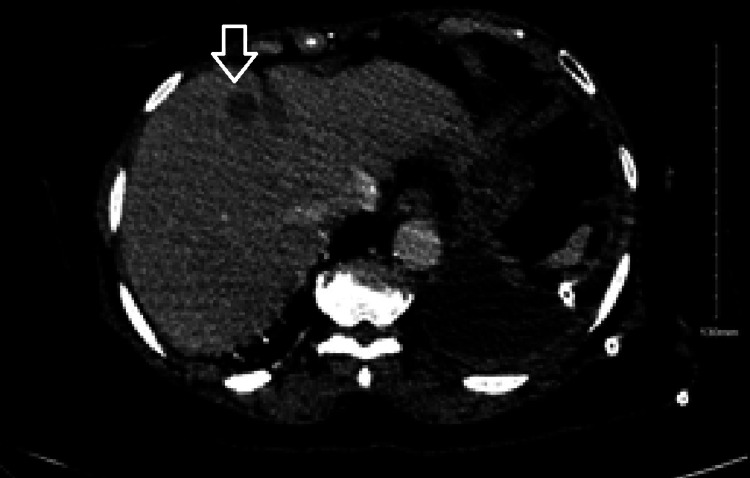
Abdominal CT demonstrating metastatic liver lesions (arrow), as seen by a hypodense lesion noted on the liver
